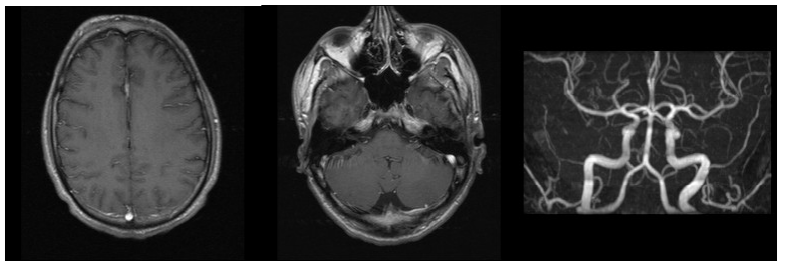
(a, b) Contrast enhanced magnetic resonance imaging revealed non-enhancing lesions. (c) Time-of-flight angiography did not detect cerebral vasculitis and showed normal vessel calibers.
